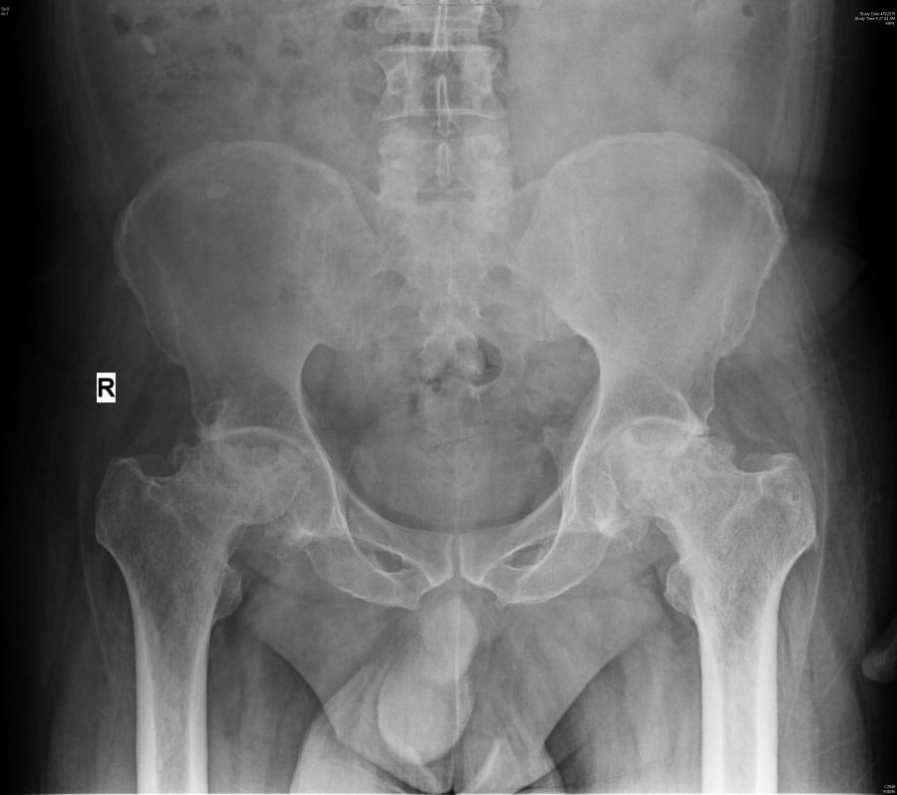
X-ray pelvis anteroposterior view showing avascular necrosis of the head of the femur
